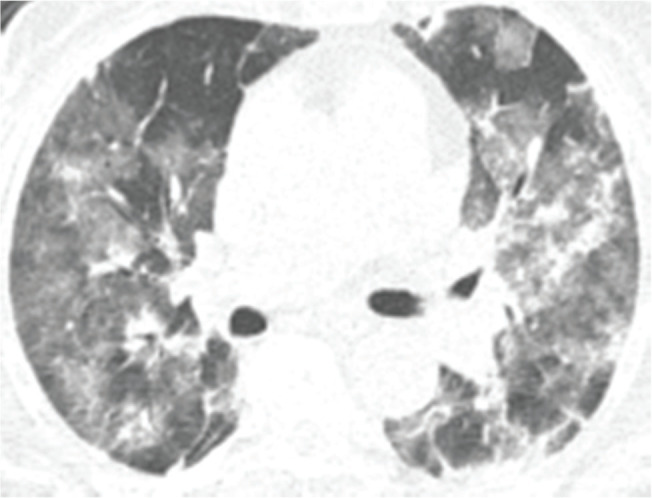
Indeterminate findings of COVID-19. Axial CT image shows bilateral diffused GGOs associated with consolidations, and some areas of septal thickening.
